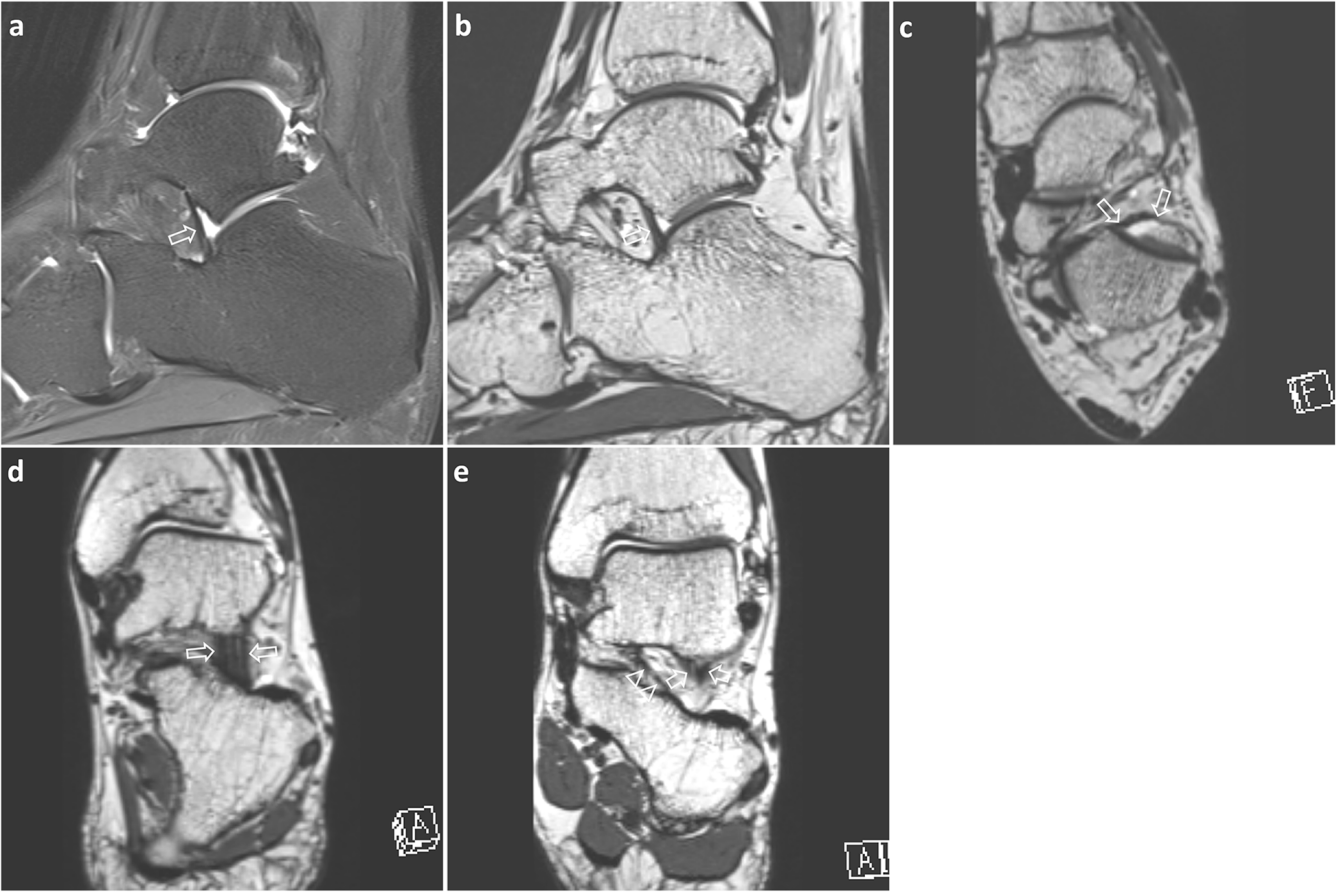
A 29-year-old man with posttraumatic soft tissue functional impingement. Sagittal T2-weighted image with fat suppression (a) demonstrates uniform thickness of band-shaped IOLV (arrow) before posterior talocalcaneal joint. Sagittal (b), axial (c) and oblique coronal (d) 3D isotropic T2-weighted images represent IOLV thickness and width (arrows), starting from the anterior margin of the posterior facet of the talus and extending vertically across the subtalar joint. In the coronal (e) 3D isotropic image along the posterior wall of the tarsal sinus, IOLO (arrowheads) courses obliquely from the inside, and IOLV (arrows, partially visualized) runs vertically further outward. IOLV, interosseous ligament vertical segment; IOLO, interosseous ligament oblique segment
